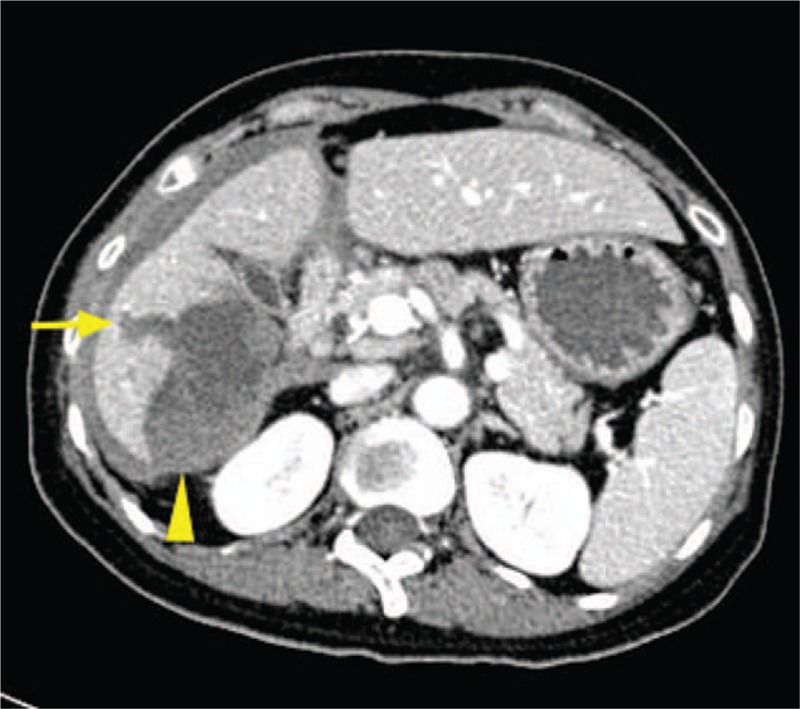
Contrast-enhanced computed tomography (CECT) at the Department of Emergency. CECT demonstrated liver rupture (arrow) at the right inferior segment of the liver with subcapsular hematoma (arrowhead).
